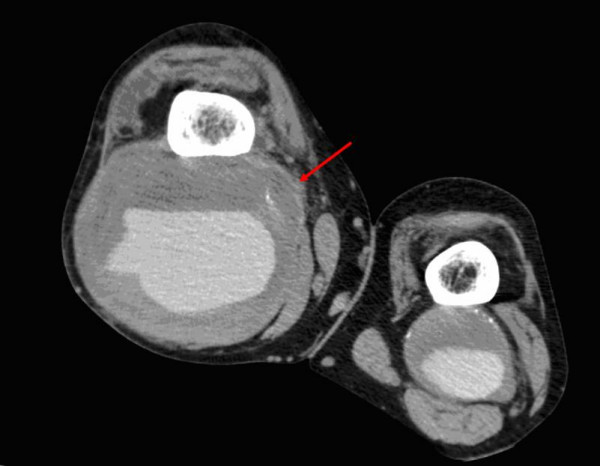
CT image showing bilateral popliteal artery aneurysms with extravasation of contrast-enhanced blood with surrounding aneurysm on the right side. (Arrow pointing to the popliteal artery).
